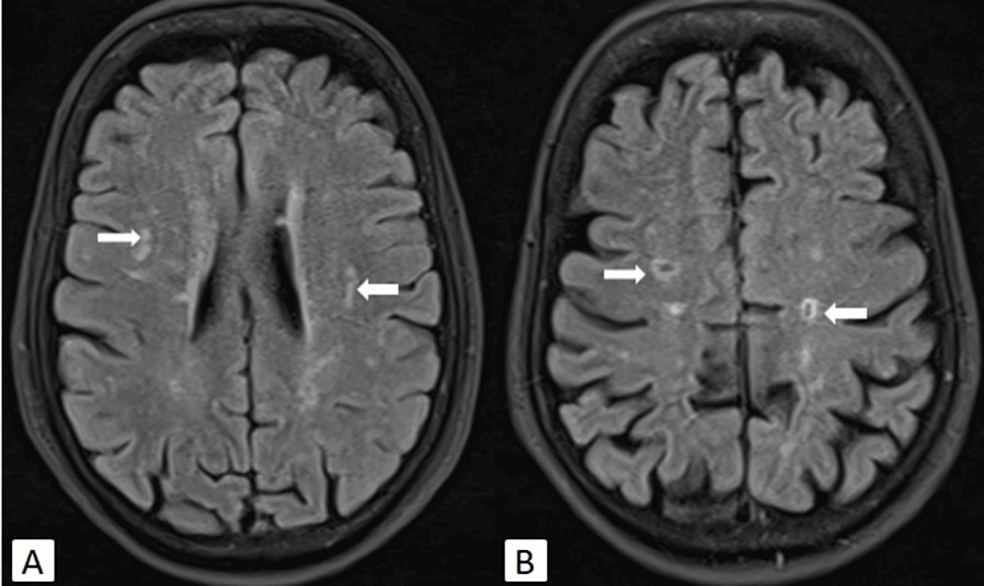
MRI of the brain axial FLAIR images showing white matter ischemic changes (A) and old lacunar infarcts (B) in the bilateral periventricular white matter and centrum semiovale region (white arrows). FLAIR: fluid-attenuated inversion recovery; MRI: magnetic resonance imaging
